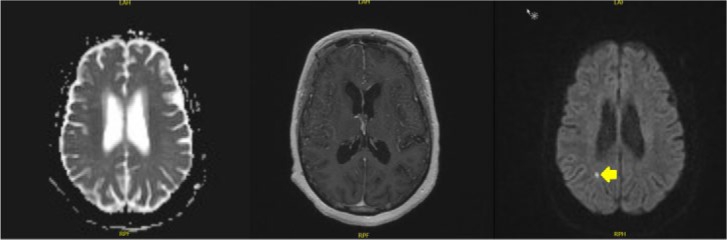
MRI at admission. Marked improvement in leptomeningeal/pial enhancement with mild residual enhancement (in the posterior fossa). Mild diffuse volume loss with stable prominence of the ventricular system. Five millimeter punctate focus of white matter restricted diffusion in the right parietal lobe.
